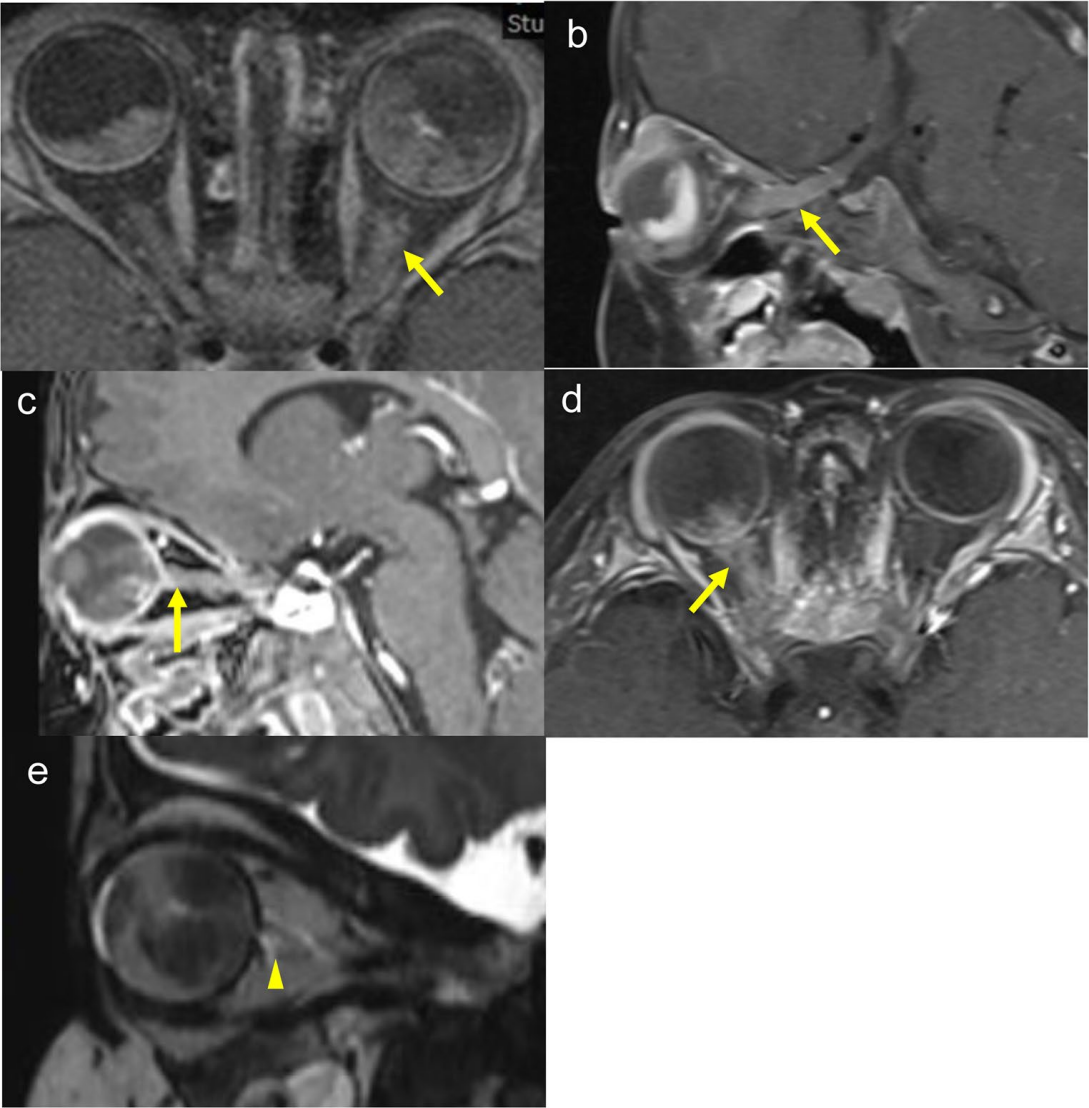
Contrast magnetic resonance imaging (MRI) images of case 1–4 (a-d) and MRI SPACE image of case 5 (e). ON contrast enhancement shows ON invasion (arrows). An enlarged origin of the left ON with disruption of the surrounding subarachnoid space indicates ON invasion (arrowhead)
